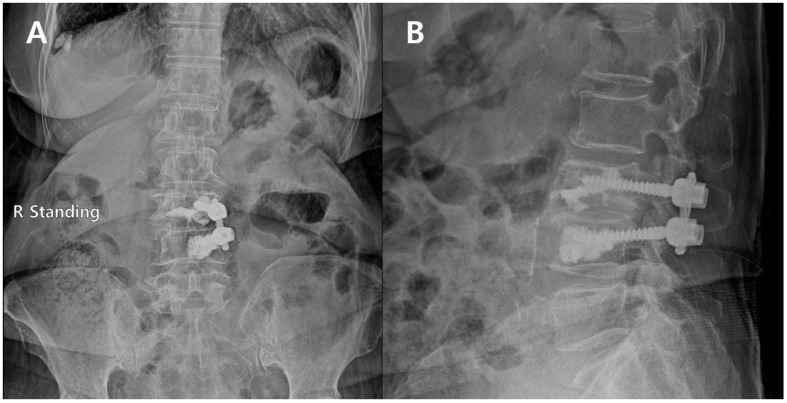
Postoperative L-spine X-ray shows cement-augmented mono screw fixation. (A) AP view on X-ray. (B) Lateral view on X-ray.
